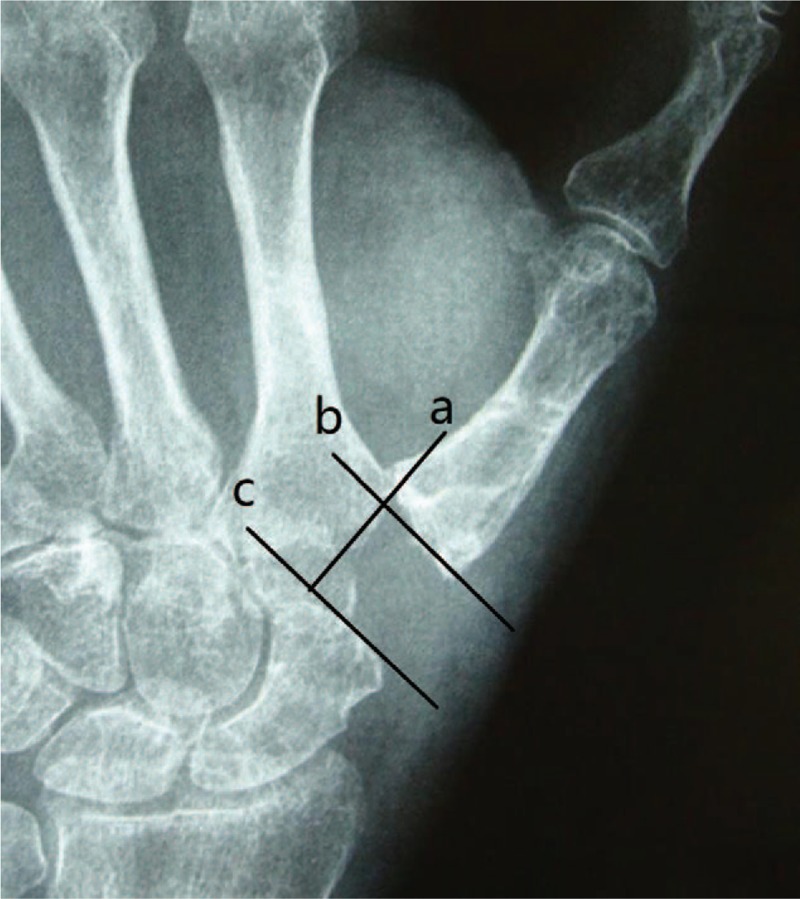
Hand X-ray showed that height of the trapezial space at 2-year follow-up. Radiographic reference points for measurement of the height of the CMC space. Line (a) is the line projected through the radial articular surface of the index metacarpal the trapezium. Line (b) is the line tangent to the thumb metacarpal base and perpendicular to line (a). Line (c) is the line tangent to the distal extreme of the scaphoid and perpendicular to line (a). The distance between line (b) and line (c) is the height of the CMC space. CMC = carpometacarpal.
